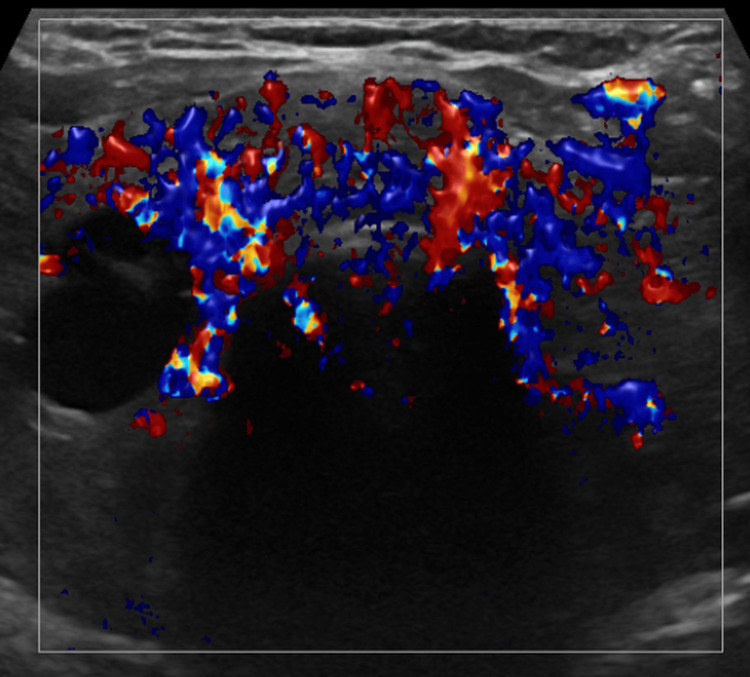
Ultrasound of the thyroid showing diffusely enlarged and heterogeneous thyroid lobes with increased vascularity, consistent with Graves’ disease
